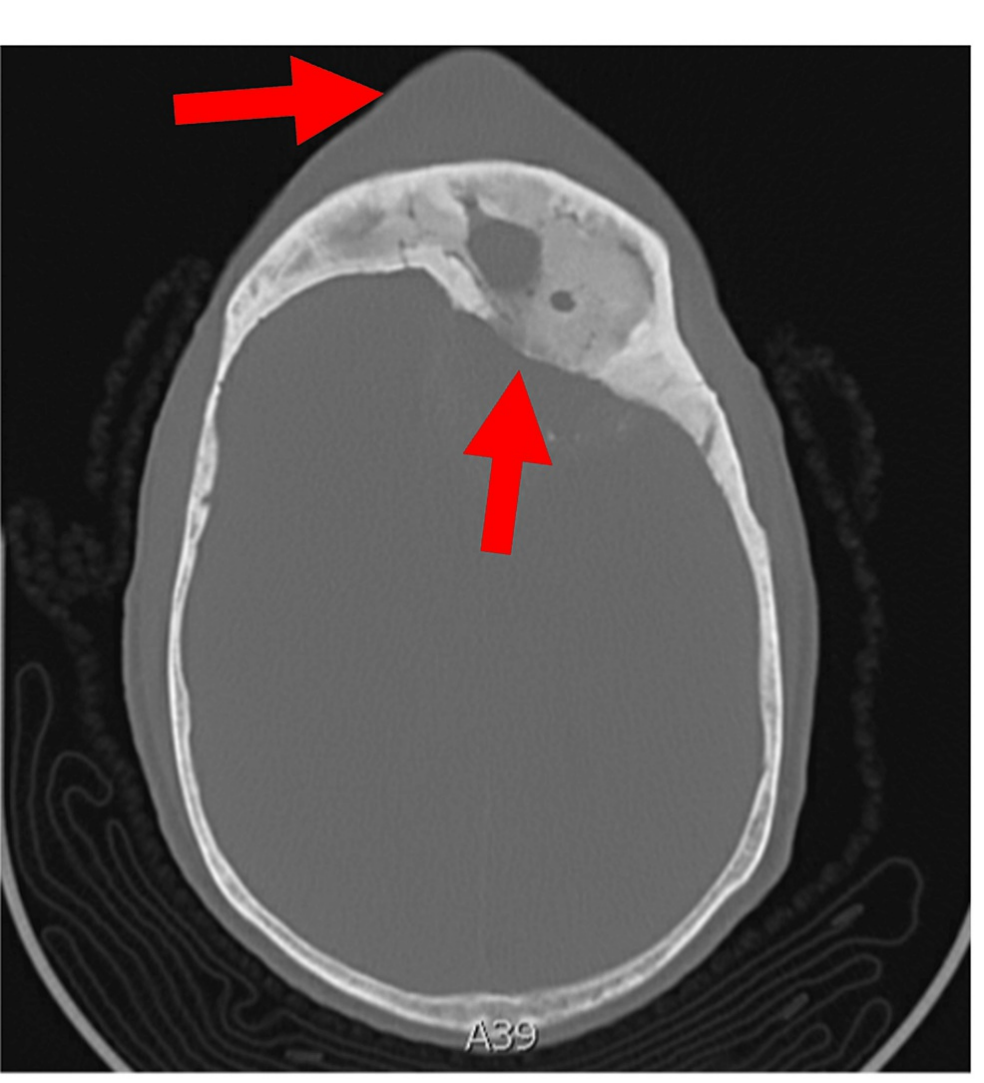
Transverse View of CT Head
